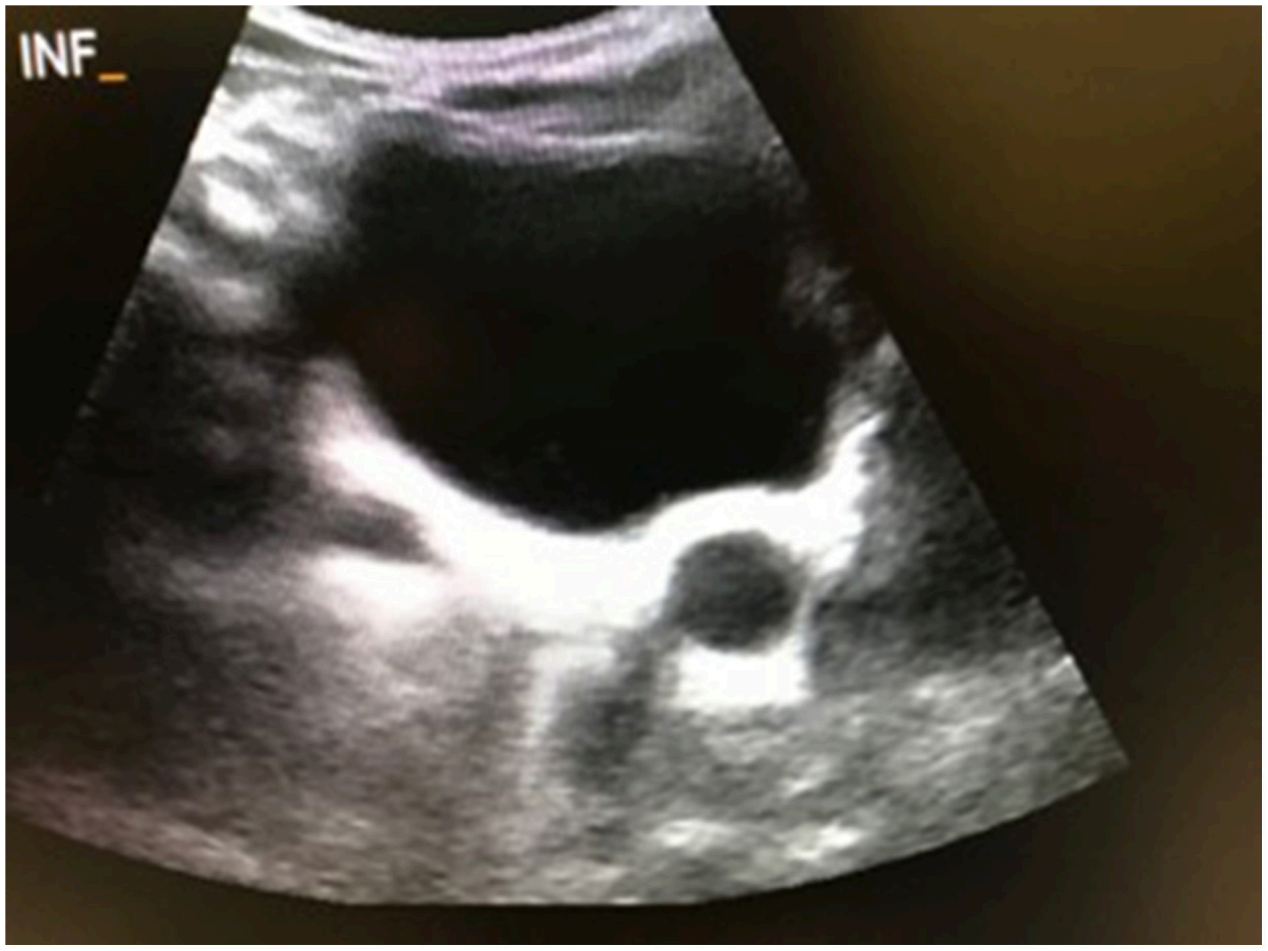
Transverse ultrasound view of bladder with dilated ureter.
